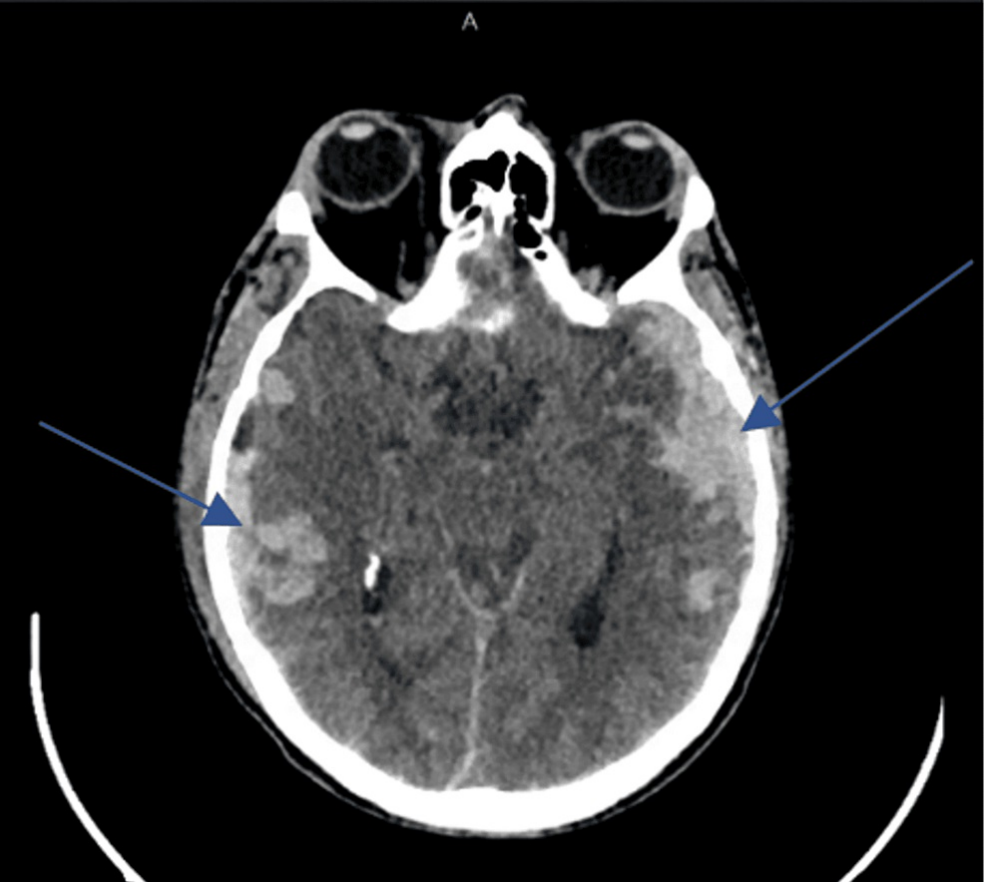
Head CT demonstrating bilateral subdural hematoma in the right and left temporal lobes (blue arrows)
